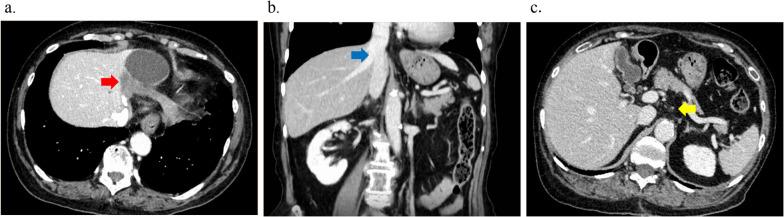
Radiological examinations after neoadjuvant chemotherapy (NAC). a, b The size of the tumor has decreased to 18 mm, and the tumor is no longer attached to the IVC (a axial view of CT; b coronal view of CT). c Lymph node enlargement has almost disappeared (axial view of CT)
